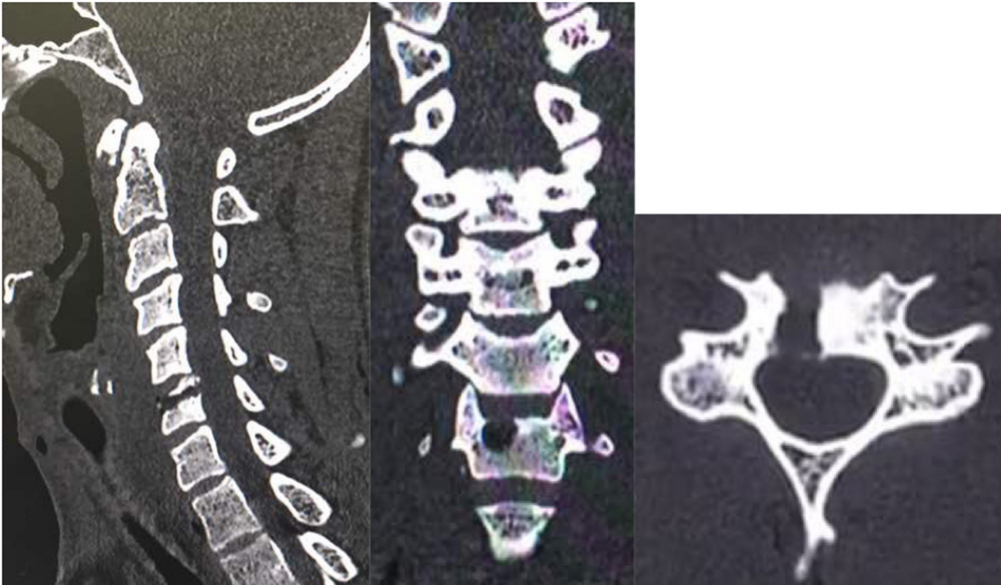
Postoperative CT scan demonstrating adequate excision with preservation of bony end plate.
